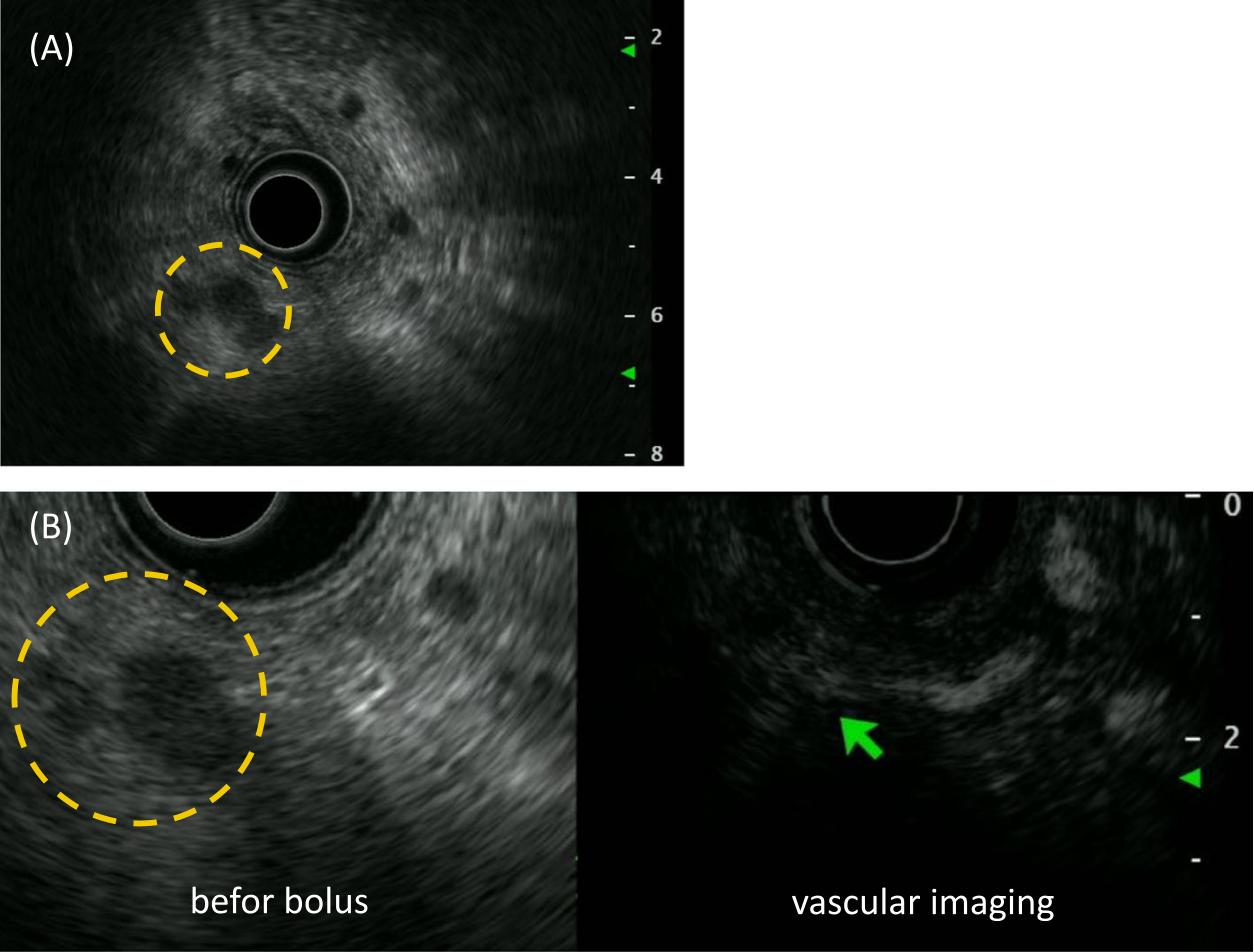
Endoscopic ultrasound findings. a Endoscopic ultrasound showed a low-density mass measuring about 16 mm in size in the pancreatic tail (circle). b The low-density mass in the pancreatic tail was partially enhanced on the contrast-enhanced ultrasound with bolus administration of Sonazoid
